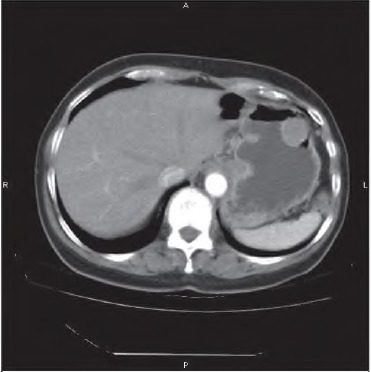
Computed tomography showing a 2cm submucosal lesion in the mid body of the stomach
